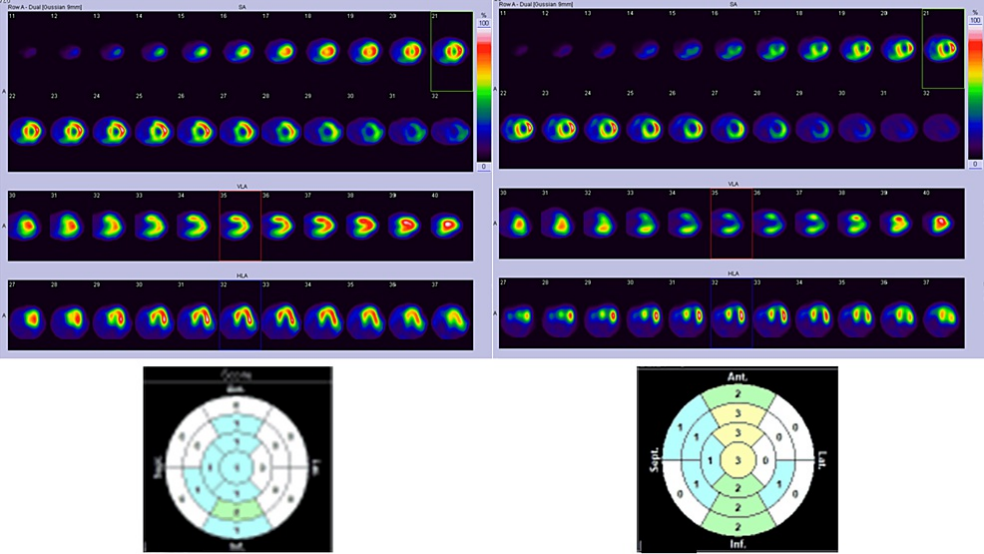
Tl/BMIPP dual-isotope myocardial scintigraphy of Case A. Top left: ^201^Tl myocardial SPECT. Top right: ^123^I-BMIPP myocardial SPECT. Bottom left: Heart Risk View-S scores for ^201^Tl uptake reduction displayed in 17-segments map. Bottom right: Heart Risk View-S scores for ^123^I-BMIPP uptake reduction displayed in 17-segments map. SPECT, single photon emission computed tomography; ^123^I-BMIPP, ^123^I-β-methyl-P-iodophenyl-pentadecanoic acid
